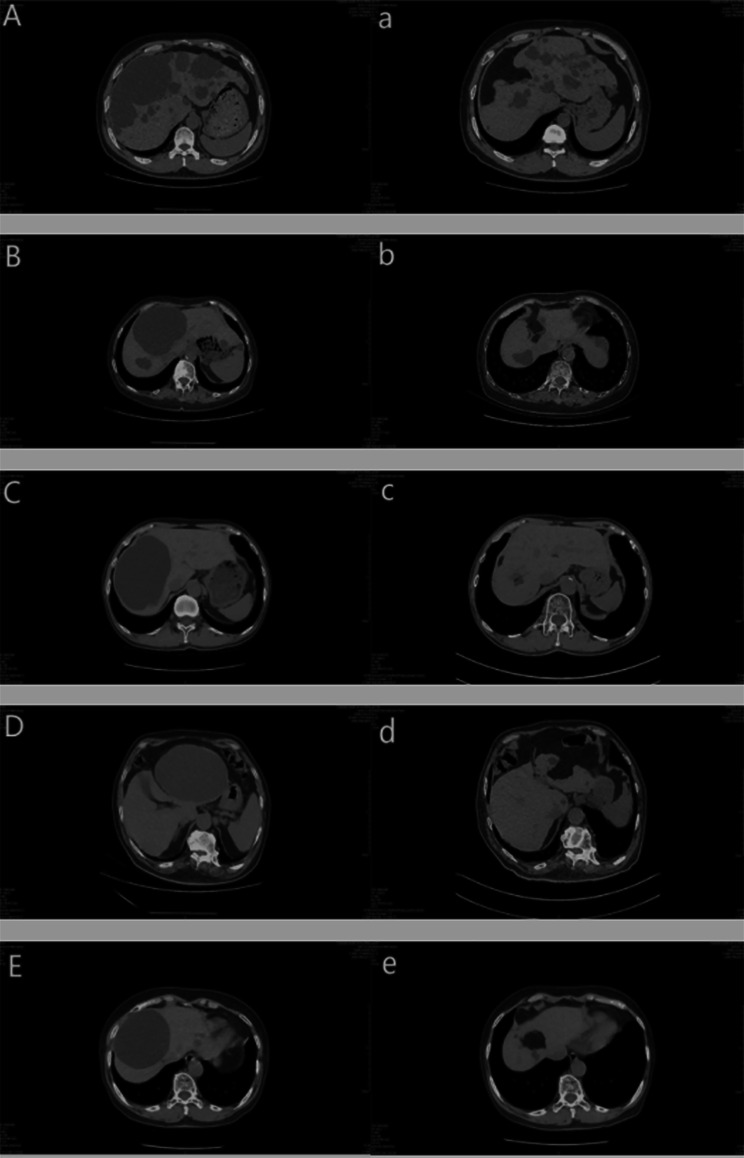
Imaging comparison between preoperative and 12 months after surgery A,B,C,D,E Location and size of hepatic cysts revealed by preoperative liver CT imaging a,b,c,d,e In the liver CT image 12 months after fenestrating hepatic cyst combined with pedicled greater omentum flap tamponade, it was found that the old hepatic cyst cavity was completely filled with pedicled greater omentum flap, and the greater omentum flap was completely anastomosed with the wall of hepatic cyst, and there was no recurrence of hepatic cyst in the operative area
